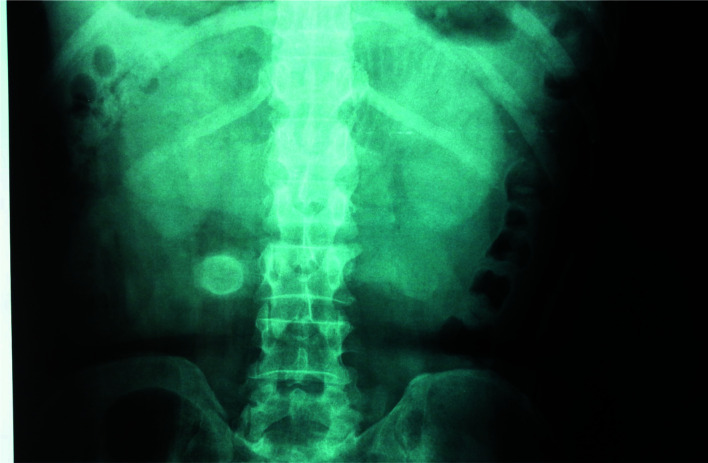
Plain x-ray showing radio-opaque object in the right lower quadrant of the abdomen.
